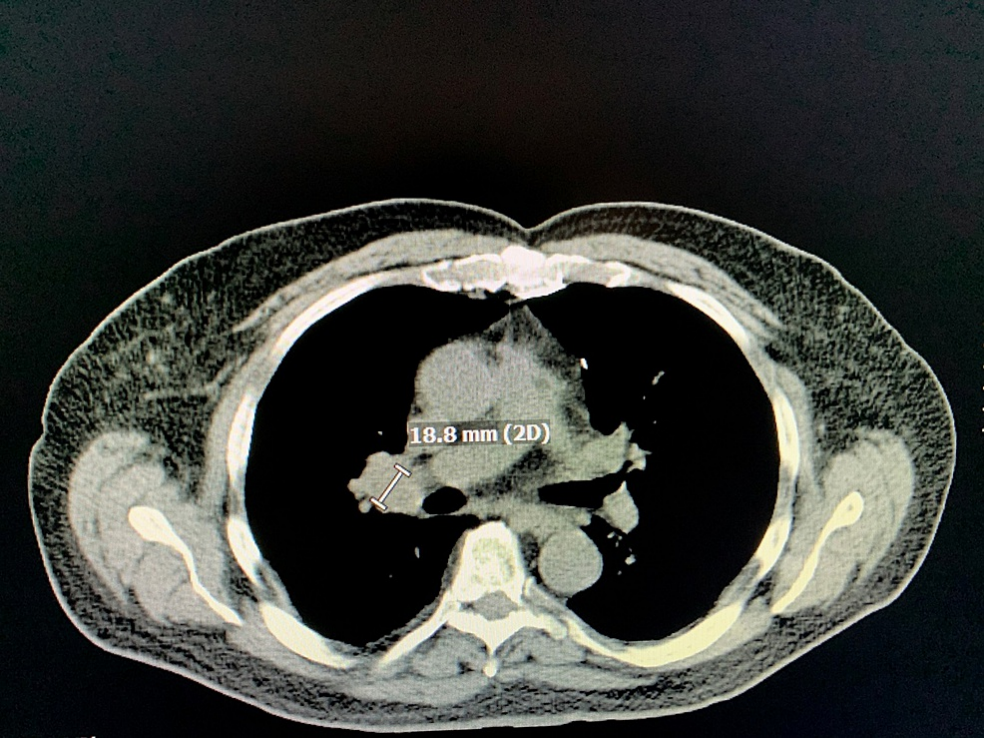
CT scan showing right hilar mass
